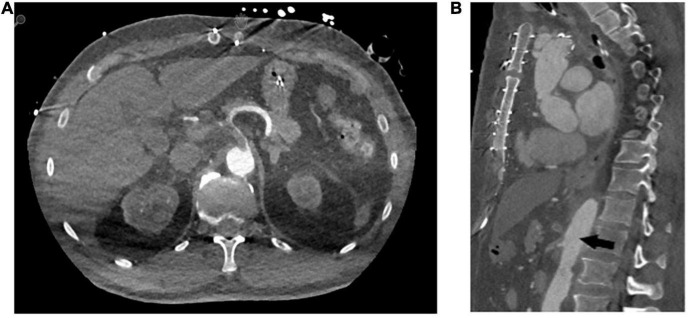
Representative computed tomography angiography images of distal embolisation of the in-stent thrombi to the coeliac trunk (A) axial plane (B) sagittal plane.
